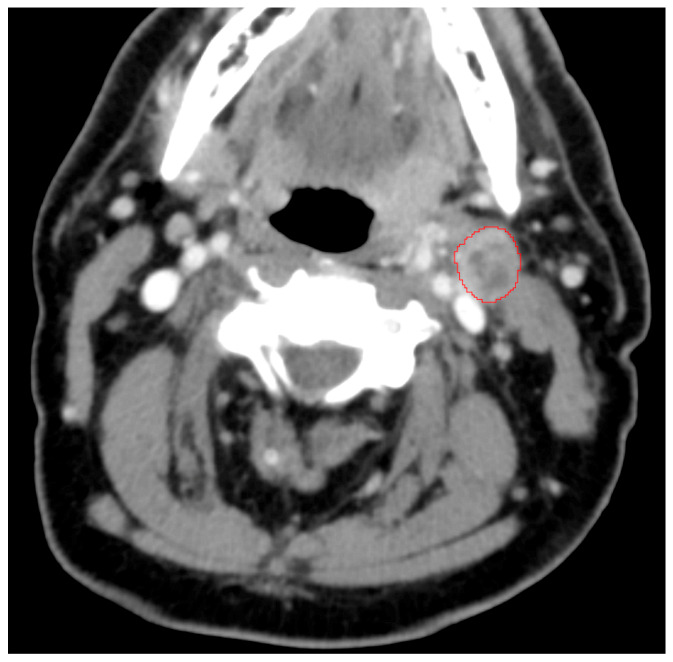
An enlarged lymph node and region of interest outlined on a pretreatment axial CT image.
